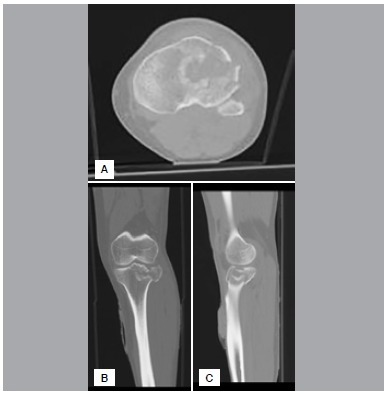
(A) Preoperative horizontal CT view; (B) preoperative frontal CT view; (C) preoperative sagittal CT view.
